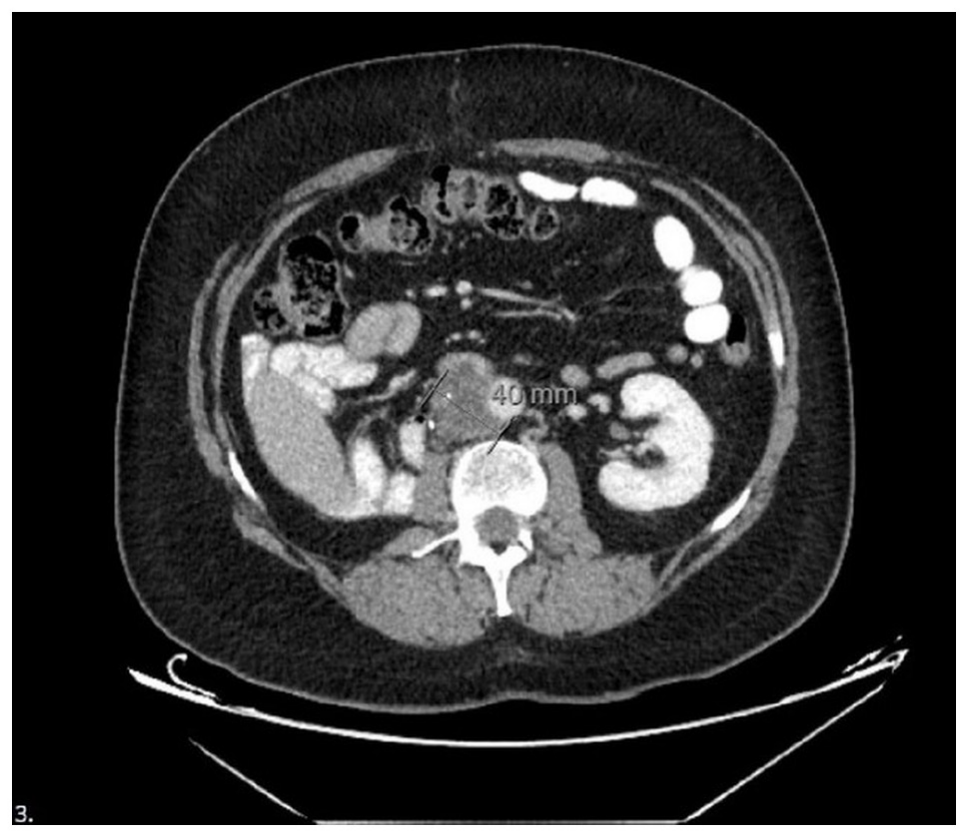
Computed tomography image of the retroperitoneal mucinous adenocarcinoma lesion arising from a teratoma. Contrast-enhanced axial CT image demonstrating a heterogeneous retroperitoneal mass with cystic and solid components, consistent with teratoma-derived adenocarcinoma following chemotherapy for relapsed testicular germ cell tumor. In this CT image, the tumor along with a 40-mm lymphadenopathy (LAP) complex that was developed as a result can be observed.
